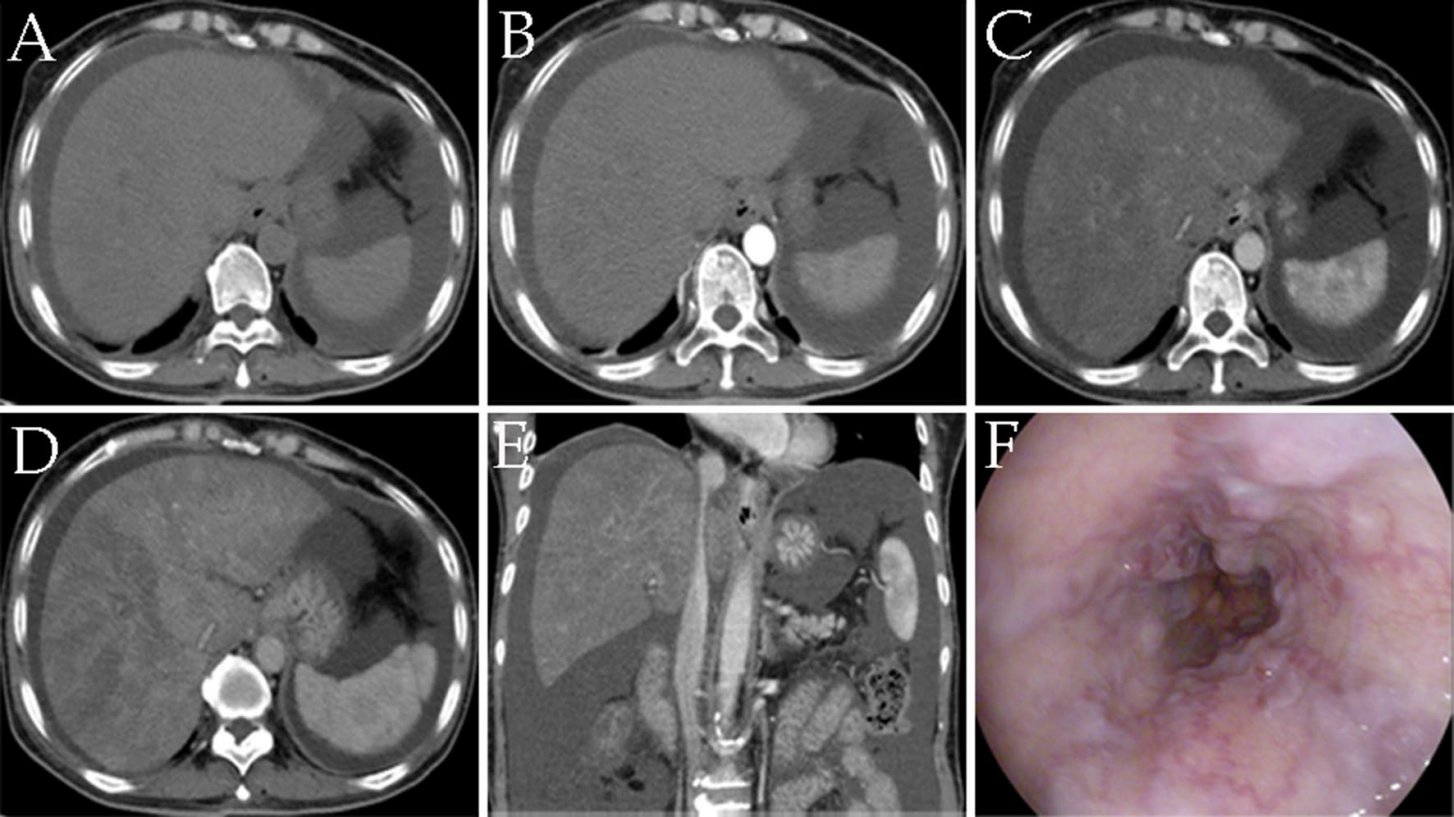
A 61-year-old woman diagnosed with pyrrolizidine alkaloid-induced hepatic sinusoidal obstruction received contrast-enhanced CT scan and gastroscopy. (A–D) Images from plain and contrast-enhanced CT scan. (A) Plain CT scan; (B) arterial phase; (C) portal phase; (D) equilibrium phases. (E) Multiple planar reconstruction CT showed that the hepatic segment of the inferior vena cava was compressed and thinner. (F) Gastroscopy revealed esophageal varices. *CT* computed tomography.
